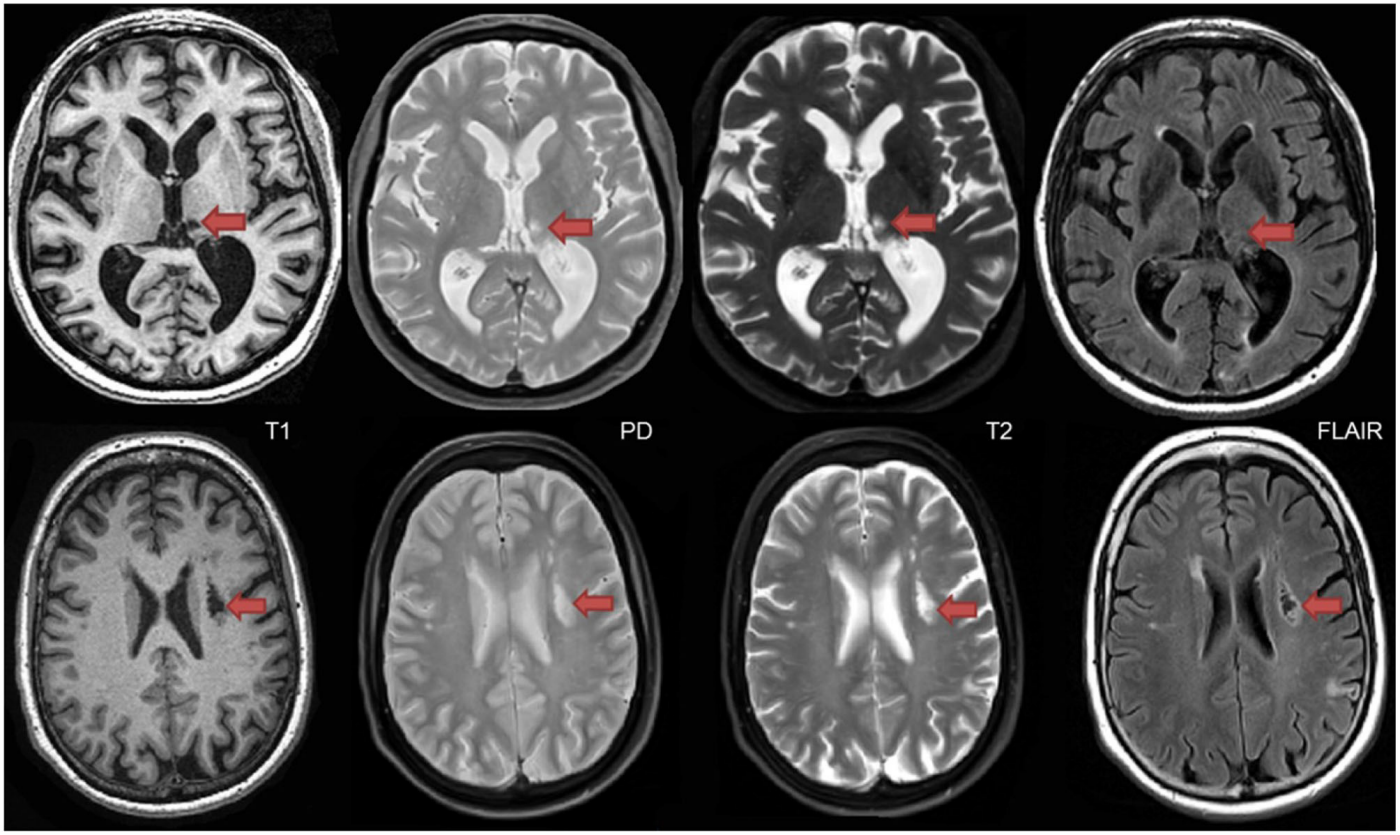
Top row shows a thalamic lacune as it appears on different coregistered MRI, hypointense (dark) on T1, hyperintense on PD-T2, and difficult to detect on FLAIR. In contrast, the bottom row shows a subcortical lacunar infarct that presents with the classic central CSF-like hypointensity with a surrounding hyperintense halo/rim on FLAIR.
